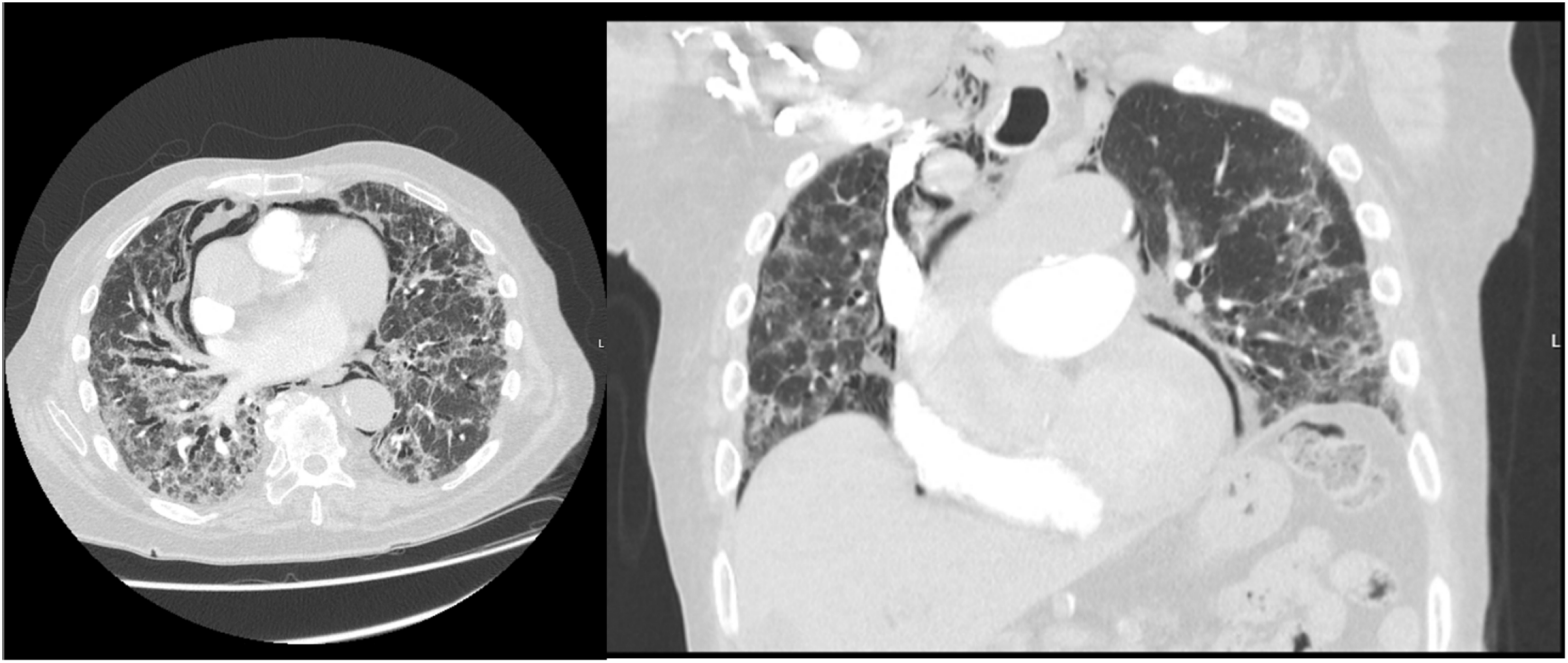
CT pulmonary angiogram demonstrating pneumomediastinum.
